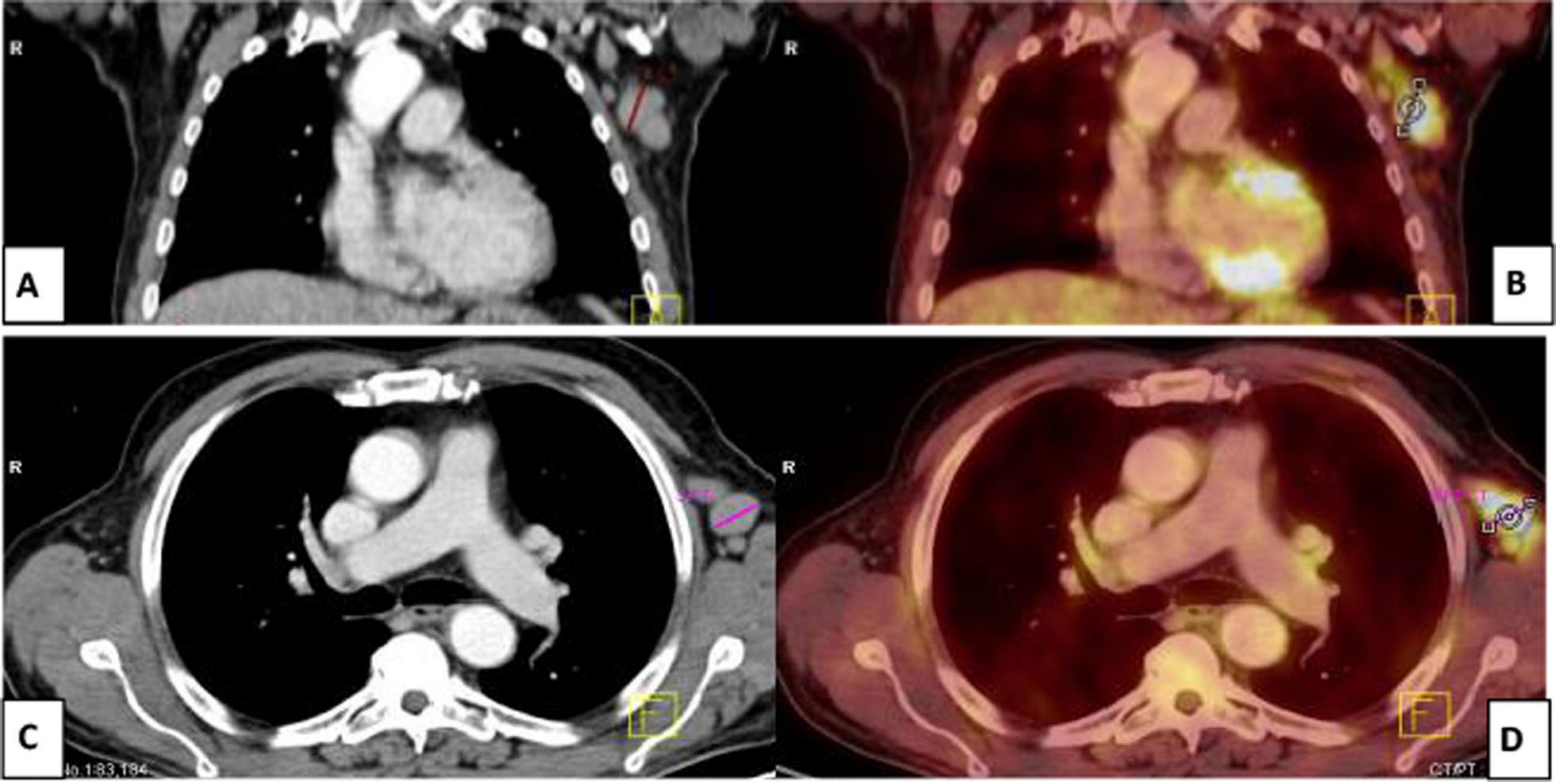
A 54-year-old male patient with Hodgkin’s lymphoma, treated with anti-cd20 antibody rituximab 4 months prior to vaccination. A Coronal and C axial CECT images revealed multiple enlarged left axillary LNs, largest measures 2.7 cm. B Coronal and D axial PET-CT images revealed enlarged and FDG-avid left axillary LNs of 3 SUVmax. At first, this was diagnosed as progression, ultrasound-guided biopsy-revealed reactive follicular hyperplasia with no malignancy. Clinical history revealed that he got the 2nd dose of Pfizer-BioNTech COVID-19 vaccine in his left arm 7 days before PET-CT study
